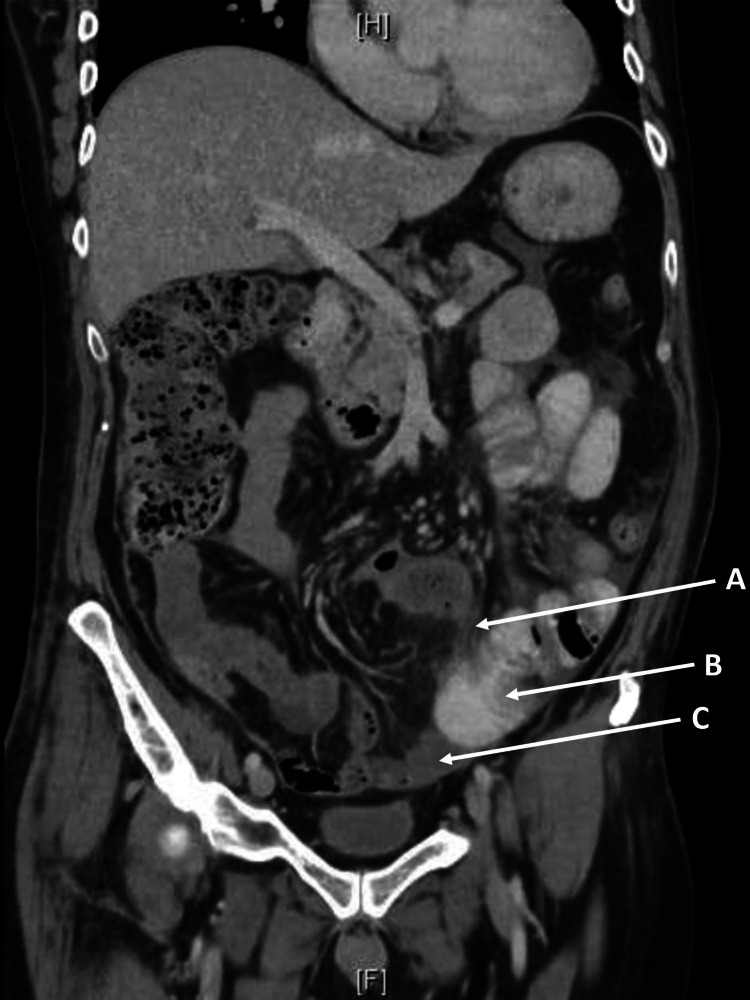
Coronal CT Abdomen and Pelvis CT abdomen and pelvis day 10 post-Hartmann’s procedure with ongoing A = mesenteric vasculitis, B = resolved colitis, and C = free peritoneal fluid.
